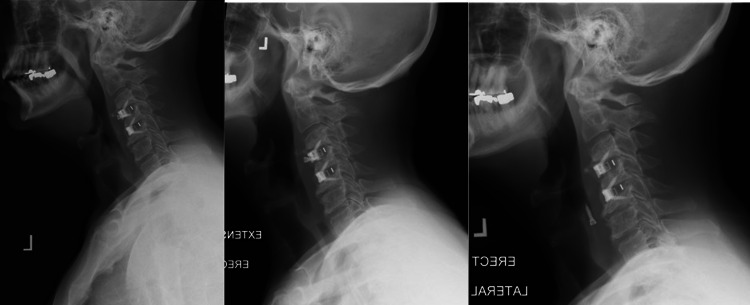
Cervical radiographic images. (A) Postoperative X-ray one year following C3-4, C4-5 ACDF. (B) Postoperative X-ray 17 months following ACDF. (C) Postoperative X-ray 18 months following ACDF. Note the caudal screw at C3-4 fusion progressively backs out until the screw is observed free-floating in the soft tissue. ACDF: anterior cervical discectomy with fusion
